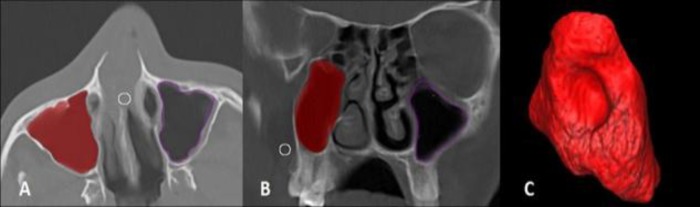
Surface area Calculation of Maxillary Sinus in axial (A) and Coronal (B) CT scans, 3D volumetric measurement of the maxillary sinus using the Amira software (C).
